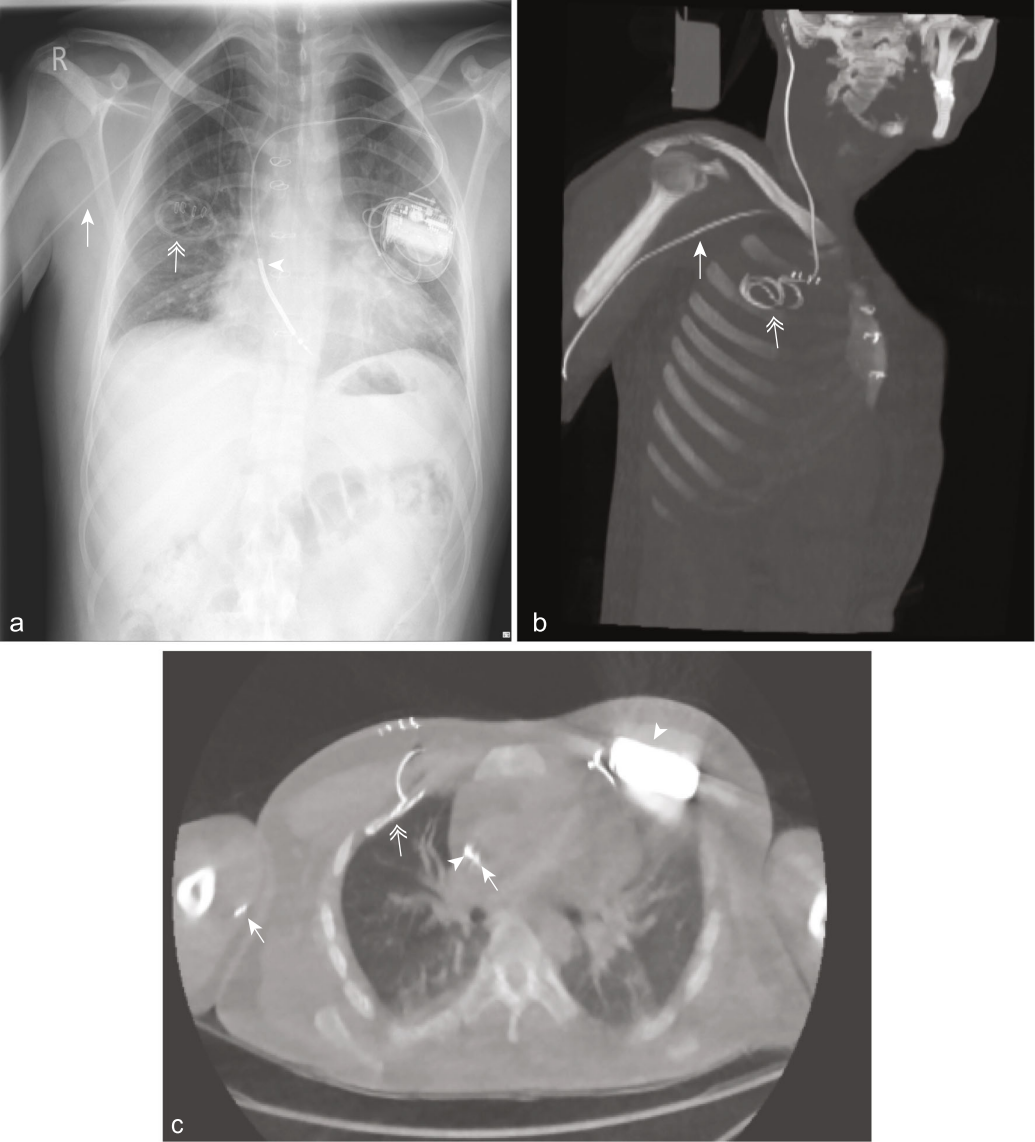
ULD CT with pure IR permits confident evaluation of shunt position postoperatively. Chest radiograph showing the distal end of a newly inserted ventriculopleural shunt (*double arrowhead*) in the mid-zone of the right hemithorax (a). ULD CT with pure IR multiplanar reconstructions clearly demonstrating the shunt course and precise anatomical localisation of the distal catheter tip in the pleural space overlying the right upper lobe (b and c). Skin clips and subcutaneous air related to the recent procedure are seen in the right anterior chest wall. On lung window, no pneumothorax or additional postoperative complication is demonstrated (c). Previous median sternotomy, left-sided single lead implantable cardioverter defibrillator (*arrowhead*) and right-sided peripherally inserted central catheter (*arrow*) are also demonstrated. *CT* Computed tomography, *IR* Iterative reconstruction, *ULD* Ultralow dose
